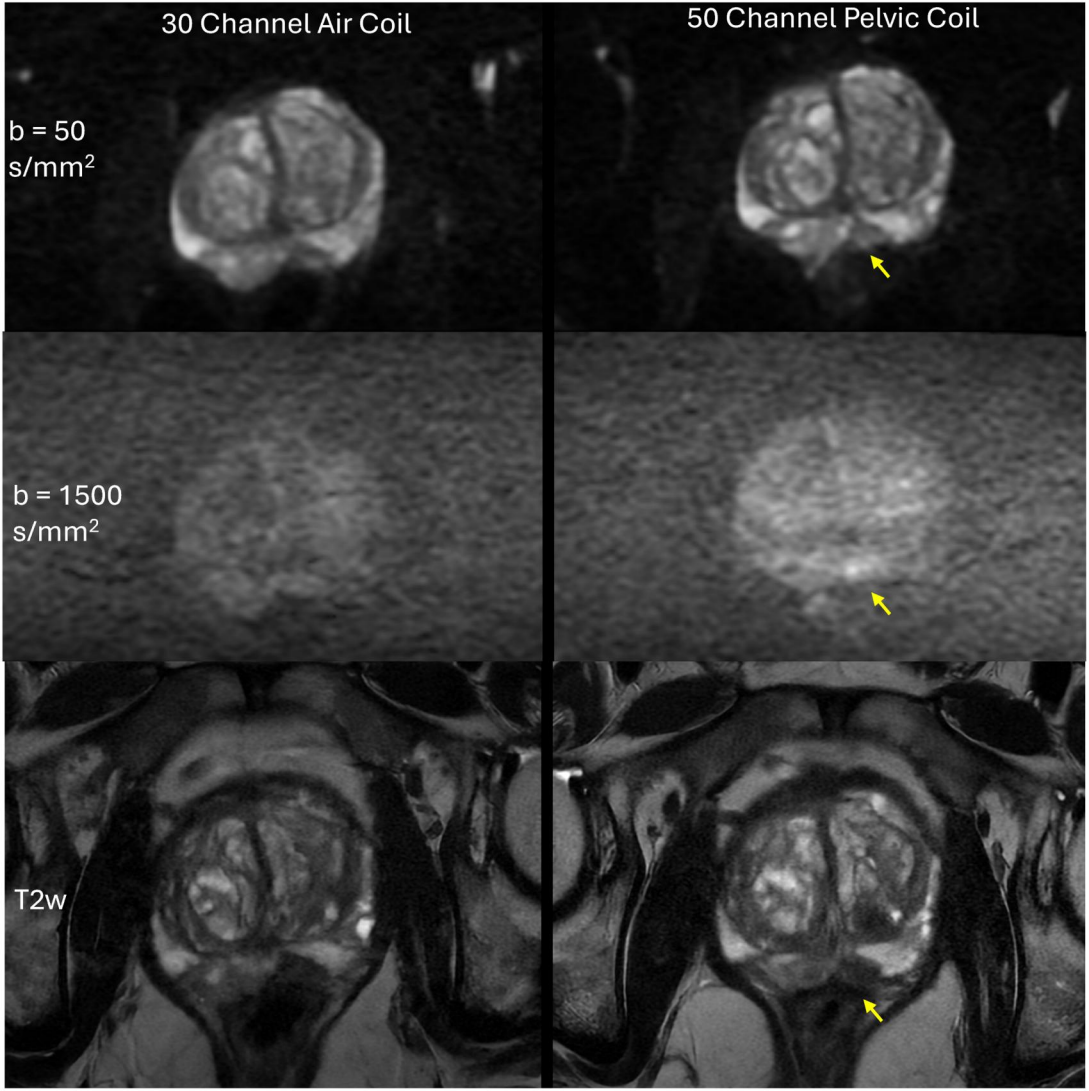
Diffusion-weighted images (DWI, *b *= 50 s/mm^2^, top; *b *= 1500 s/mm^2^, middle) and T2-weighted (T2w, bottom) images acquired using the product 30-channel AIR coil and posterior array (left) and the 50-channel pelvic coil (right) are shown. All images are reconstructed with product AIR Recon DL. This is a 71-year-old man, prostate-specific antigen = 4.72 ng/mL, with a left mid-gland posterior medial peripheral zone Gleason 3 + 3 lesion under active surveillance. Window and level are adjusted between the image pairs to ensure similar contrast despite different signal-to-noise ratio (SNR) because of coil differences. Overall, the 50-channel images have a higher perceived signal level. The *b = *50 s/mm^2^ images show better zonal distinction with the 50-channel coil. In the *b *= 1500 s/mm^2^ images, the suspected lesion (yellow arrow) has more hyperintense DWI signal with the 50-channel coil (middle, right), and the gland border is more distinct with the 50-channel pelvic coil than with the 30-channel AIR coil. Therefore, the 50-channel pelvic coil demonstrates modest enhancements in DWI. T2w images are used as an anatomical reference.
